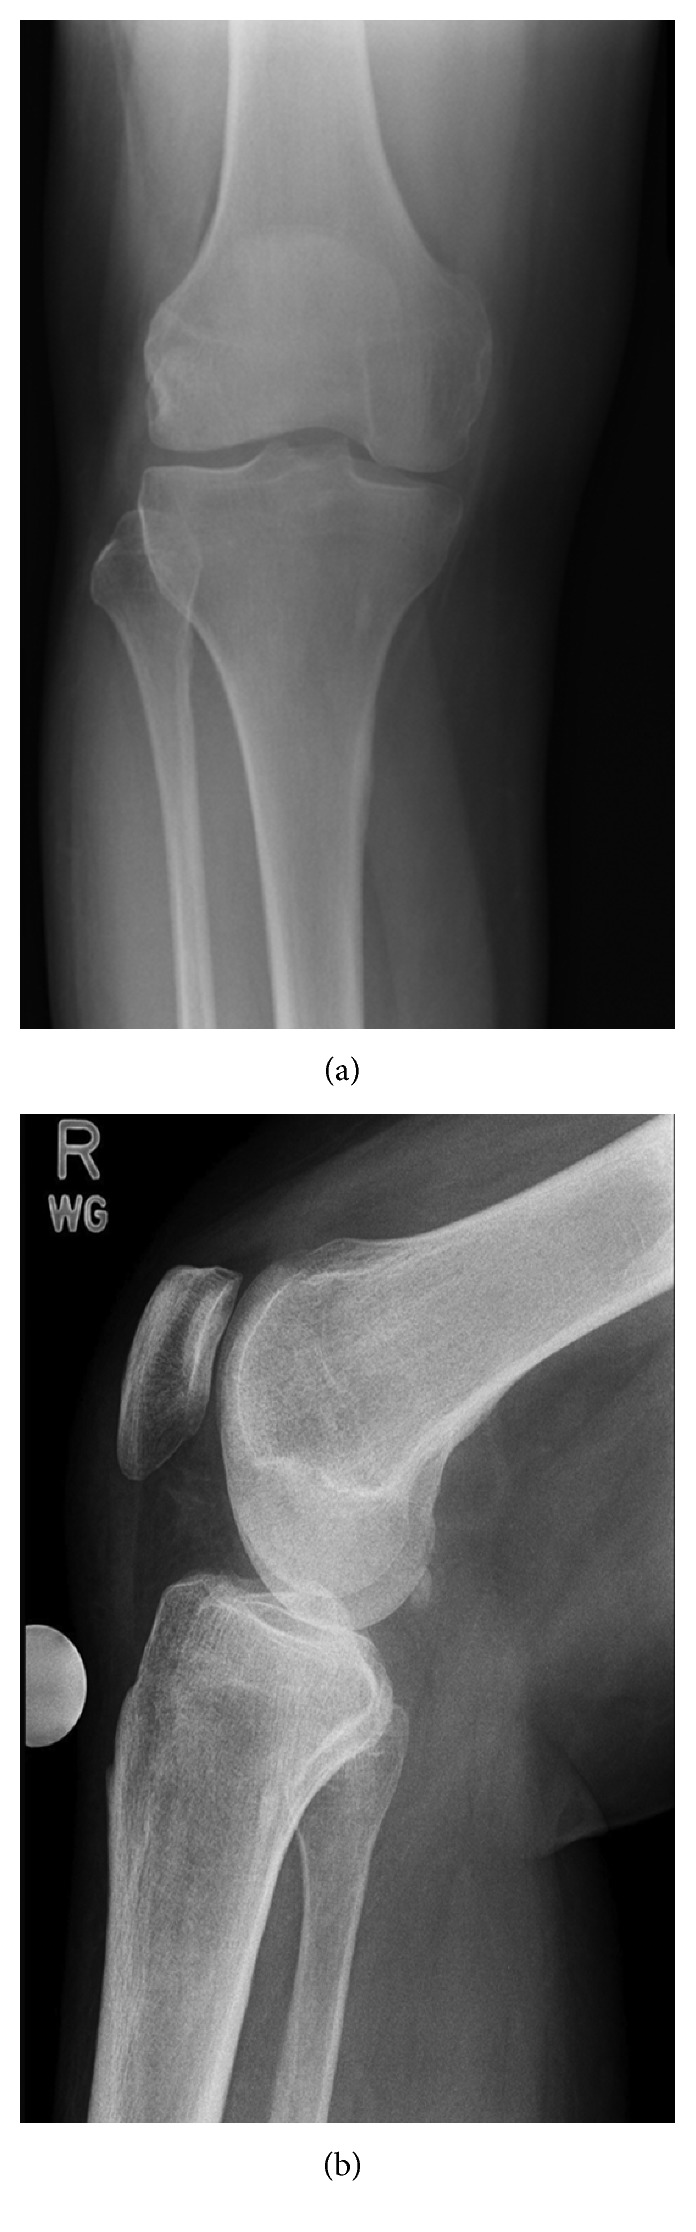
Initial (a) AP radiograph of the knee and (b) Lateral radiograph of the knee were normal.
